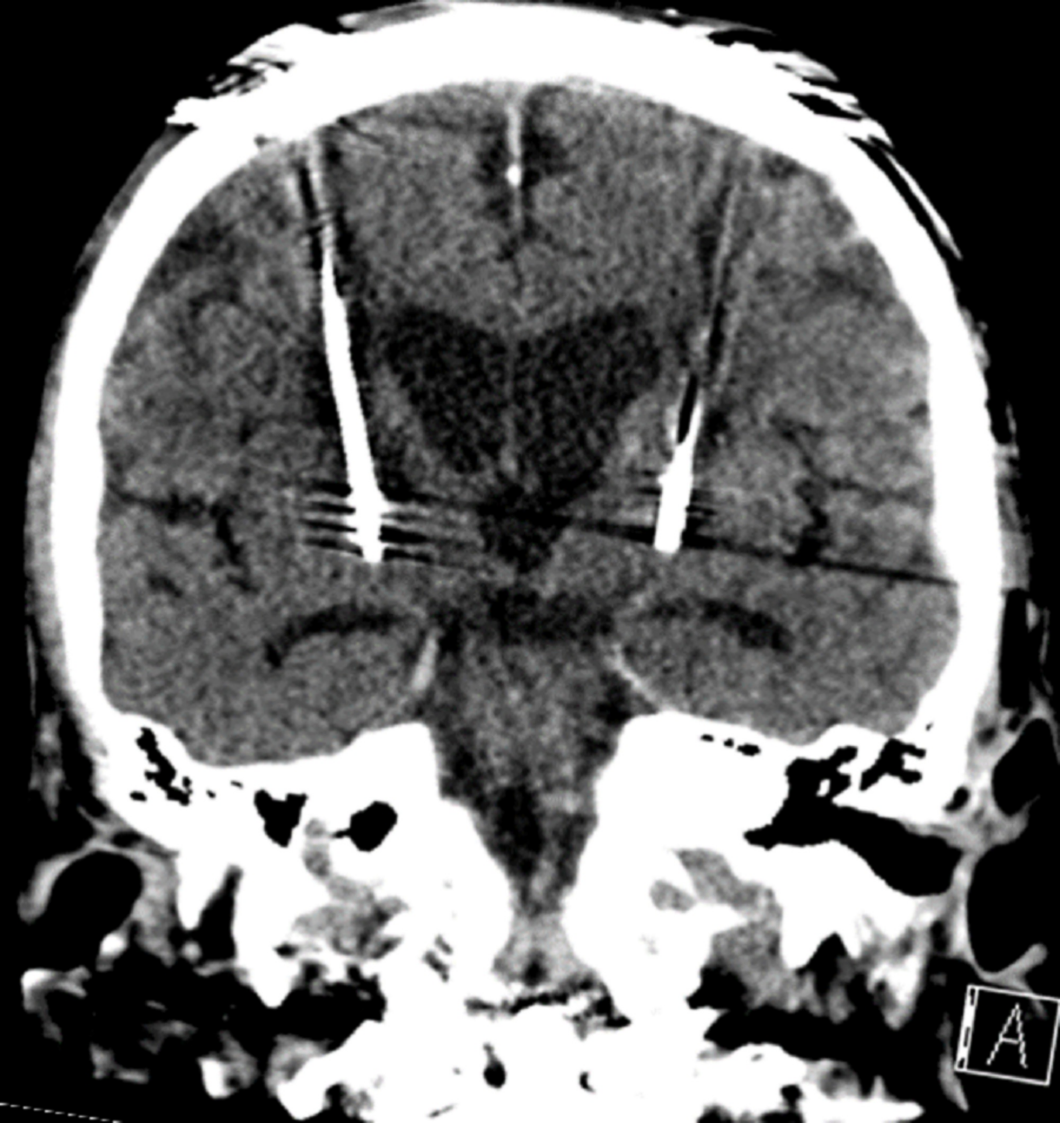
Computed tomography (CT) scan confirming placement of bilateral leads in the GPi (white arrows). GPi: Globus pallidus internus
